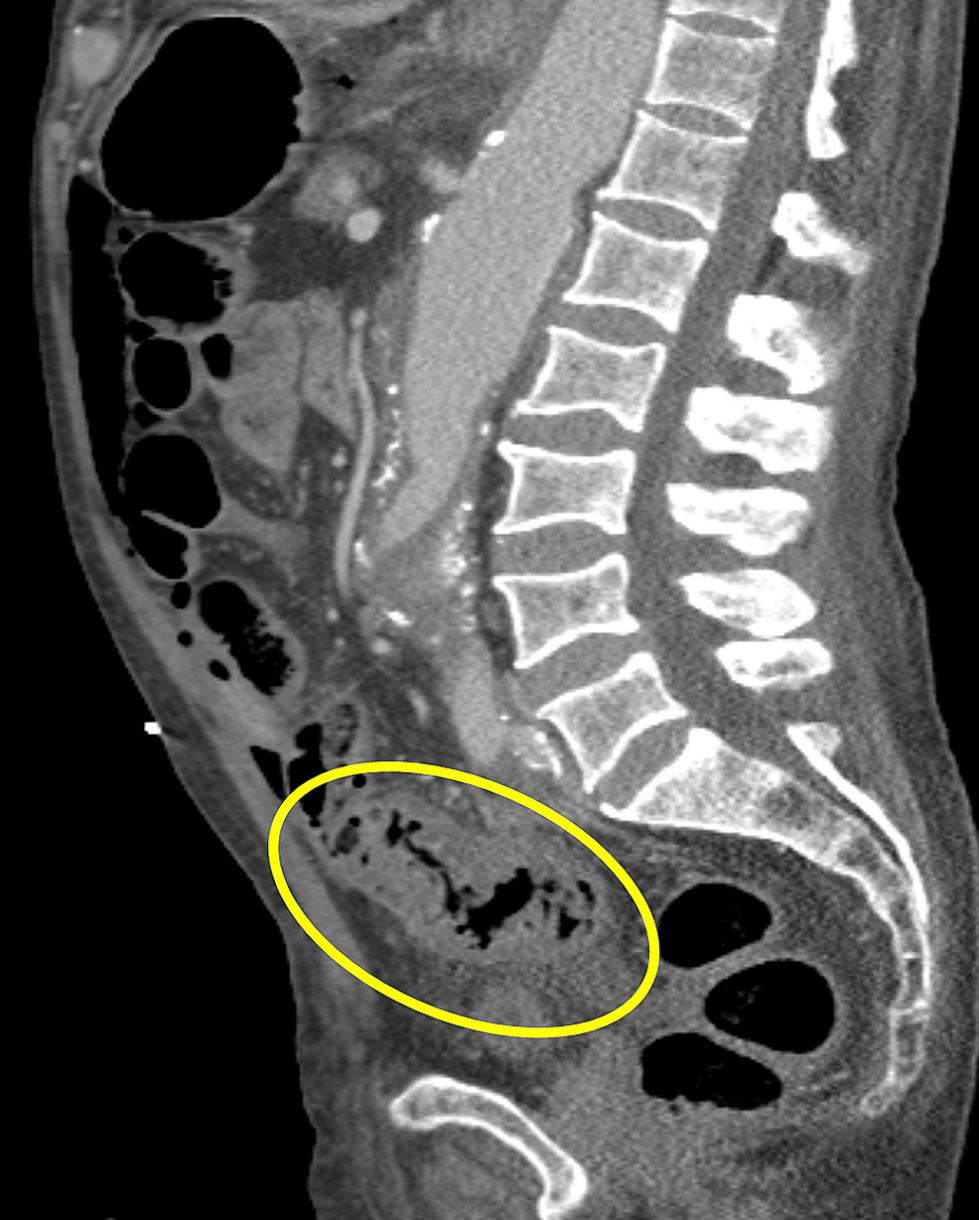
Contrast-enhanced sagittal image demonstrates diffuse circumferential thickening of the descending and proximal sigmoid colon with associated pericolonic fat stranding, in keeping with ongoing colitis. Considering the limitation due to unprepared CT scan for bowel loops evaluation, possible small intraluminal sinister polyp or mass cannot be excluded.
